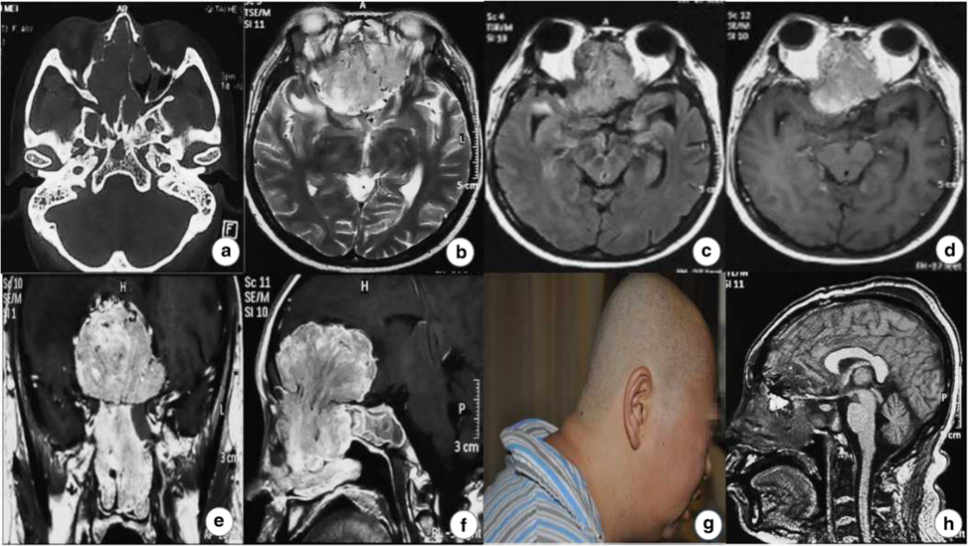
The radiological results and portraiture of the second patient. a Bone CT scans showed the neoplasm in the anterior cranial fossa eroded the bilateral sphenoid and the ethmoid sinus bone. b T2-weighted MRI showed the lesion was slight hyperintense. c The lesion showed hyperintensity in FLAIR MRI. d–f MRI scans demonstrated the obviously enhancing tumor infiltrated the nasal cavity, and some striped flow-empty action was found on the superior tumor border. g Preoperative picture showed the patient with fat centripetal distribution and pigmentation in the nape. h Postoperative MRI (1 week later) scans showed a gross removal of the intracranial tumor was achieved, but most part of it in the nasal cavity was removed
